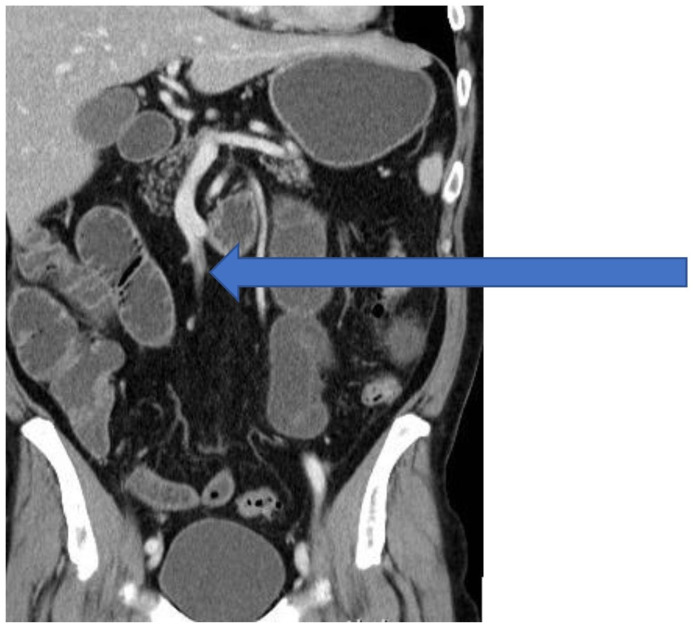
Preoperative angiographic computed tomography showed an acute completely embolic occlusion of at least three out of five branches of arteria mesenterica superior (arrow).
